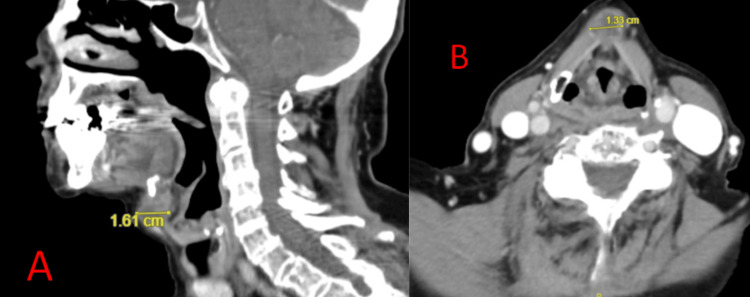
Computed Tomography (CT) Scan for Patient B Sagittal (A) and Axial (B) views from CT scan for Patient B demonstrating a midline, irregularly-shaped, cystic 1.3 cm x 1.6 cm x 2.0 cm mass just below the hyoid bone embedded within the strap muscles
